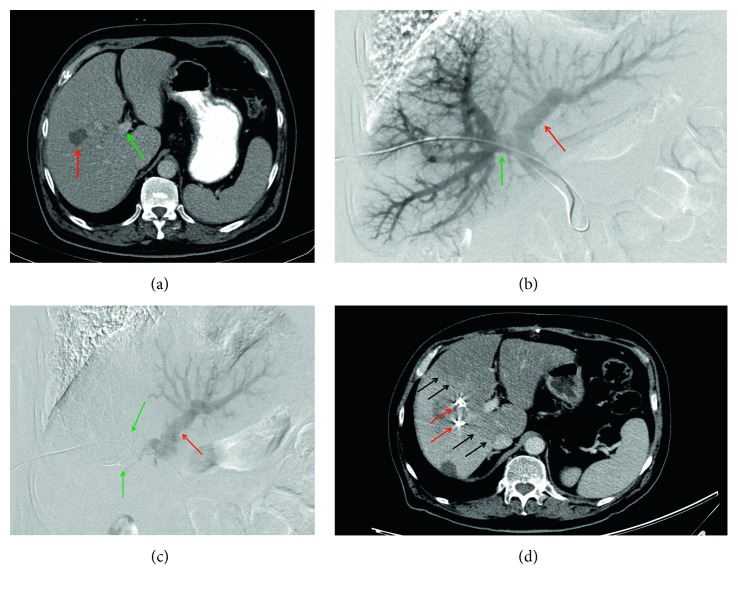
(a) A 66-year-old male with colorectal cancer presenting with right liver lobe metastasis. Computed tomography shows a small left liver (the planned surgery was a right hepatectomy), insufficient for the future right hepatectomy resection. Red arrow: liver metastasis. Green arrow: left portal vein. (b) Portography acquired immediately before portal vein embolization shows a normal portal vein anatomy. Green arrow: right portal vein; red arrow: left portal vein. (c) Portography immediately after portal vein embolization shows satisfactory occlusion of the anterior and posterior sectorial portal vein branches. Red arrow: left portal vein; green arrows: right portal branches occluded. (d) Computed tomography 4 weeks after portal vein embolization shows a significant increase in left liver volume (hypertrophy rate of 51%). Red arrows: coils placed in the right portal vein branches; black arrows: definition of the liver ischemic line between the right and left hepatic lobes.
